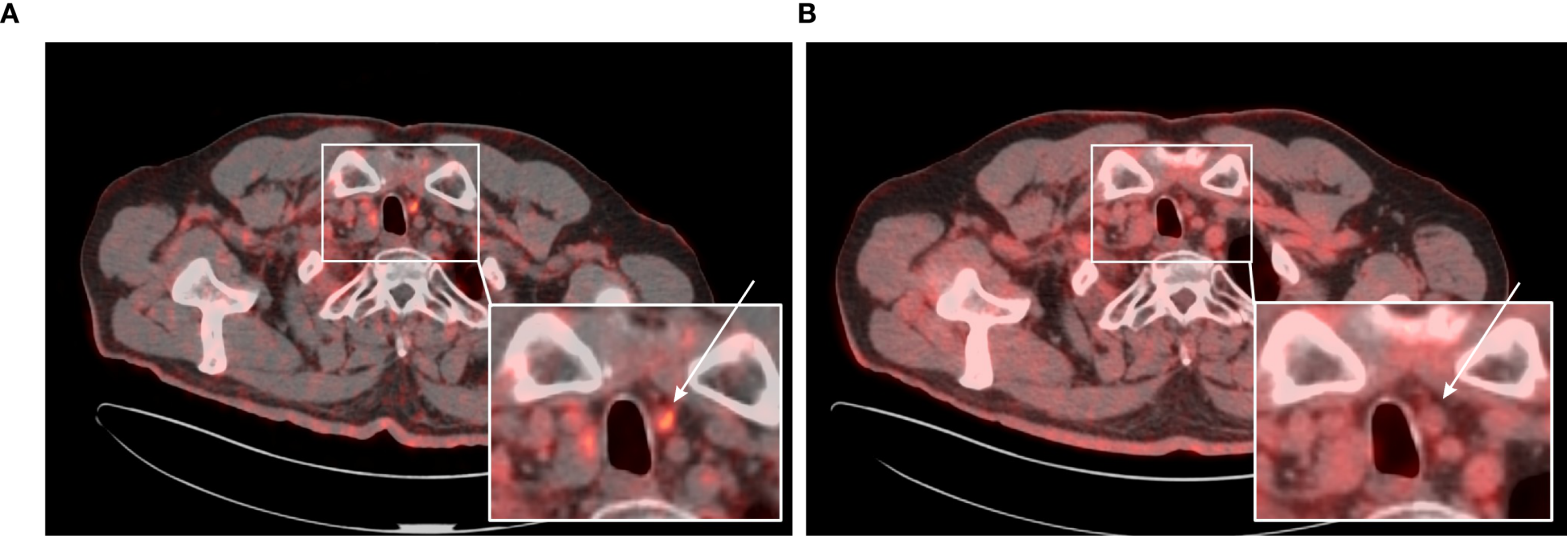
(A) 65-year-old female with medullary thyroid carcinoma and recurrence in thyroidectomy bed. (A) Transaxial view of the fused [^68^Ga]Ga-PSMA-11 PET/CT scan. (B) Transaxial view of the fused [^18^F]FDG PET/CT scan. In the thyroid bed on the left side, a spherical soft tissue lesion measuring 9 × 8 mm demonstrates increased [^68^Ga]Ga-PSMA-11 uptake (SUV_max_ 2.74; TBR_Blood_ 3.19) (white arrow). [^18^F]FDG PET/CT performed approximately 3 months later revealed that the same lesion, now measuring 11 × 10 mm, showed no evidence of increased [^18^F]FDG uptake (SUV_max_ 1.89; TBR_Blood_ 0.82) (white arrow). Fine-needle aspiration biopsy confirmed the lesion as a metastasis of medullary thyroid carcinoma.
